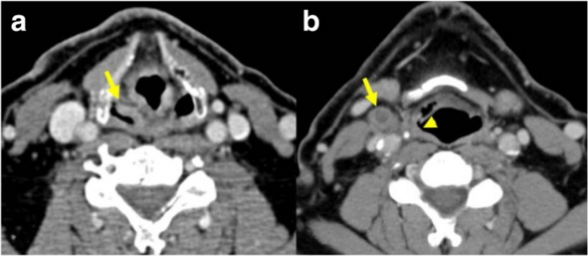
Patient with cancer of the right pyriform sinus. Contrast–enhanced CT scan a showing asymmetrical thickening of the pyriform sinus (arrow) on the right. CT scan at a lower level b showing slight thickening of the posterolateral hypopharyngeal wall (arrowhead) and an adjacent normal sized lymph node with central necrosis (arrow) indicating metastasis
